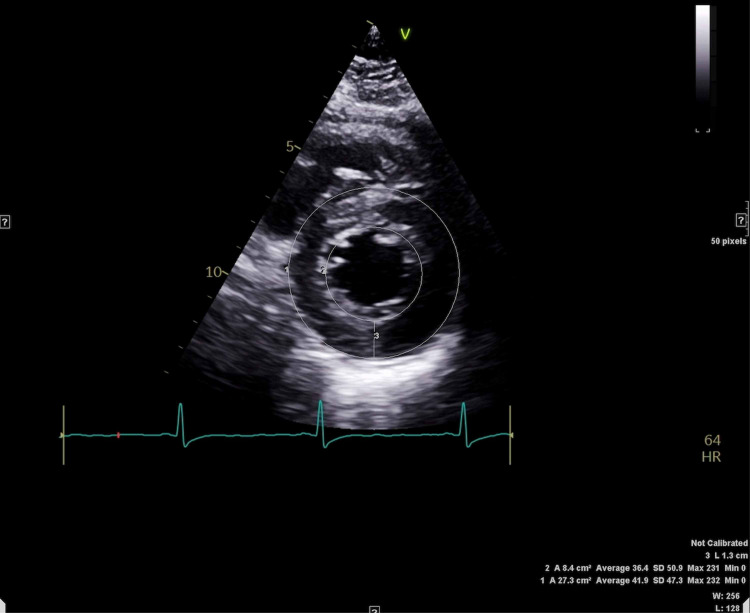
Short-axis view at level of left ventricular apex, TTE: showing left ventricle hypertrophy with concentric wall thickening up to 1.3 cm (label 3) TTE: transthoracic echocargiogram
